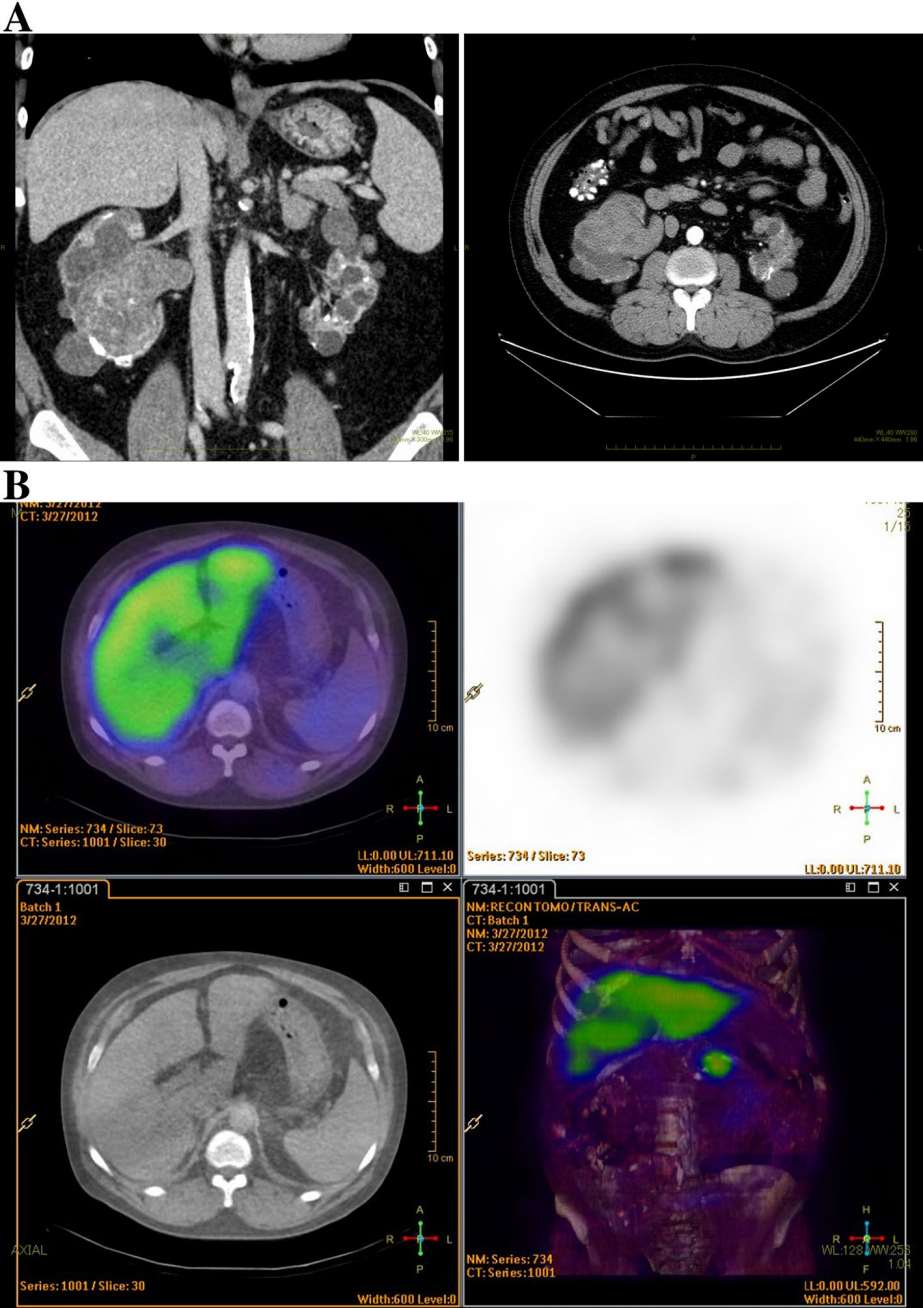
Abdominal computed tomography (A) and ^131^I-metaiodobenzylguanidine scintigraphy images (B). A Computed tomography showed multiple renal cysts in both kidneys, left kidney atrophy, and right renal pelvis tumor (64 × 55 mm). B Scintigraphy identified an increased uptake of ^131^I-metaiodobenzylguanidine corresponding to the adrenal tumor
